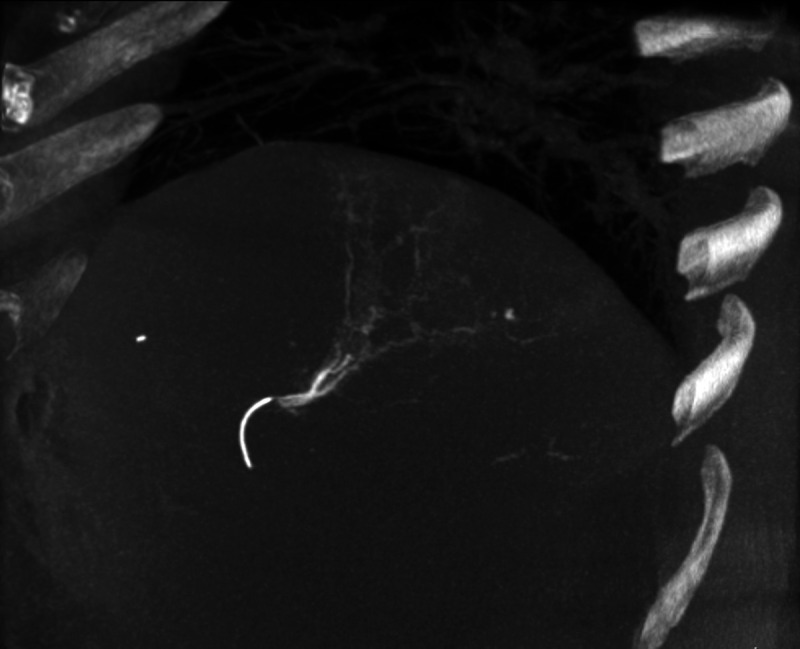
Selective cone-beam CT angiogram (sagittal plane, 50 mm MIP reconstruction) of the ^166^Ho-microspheres injection position using a microcatheter in the S VII segmental branch of the hepatic artery. The patient’s renal function remained normal on laboratory tests 1 week before and after the angiography (blood urea nitrogen: 12.6 mg/dL vs 15.7 mg/dL; creatinine: 0.72 mg/dL vs 0.74 mg/dL).
